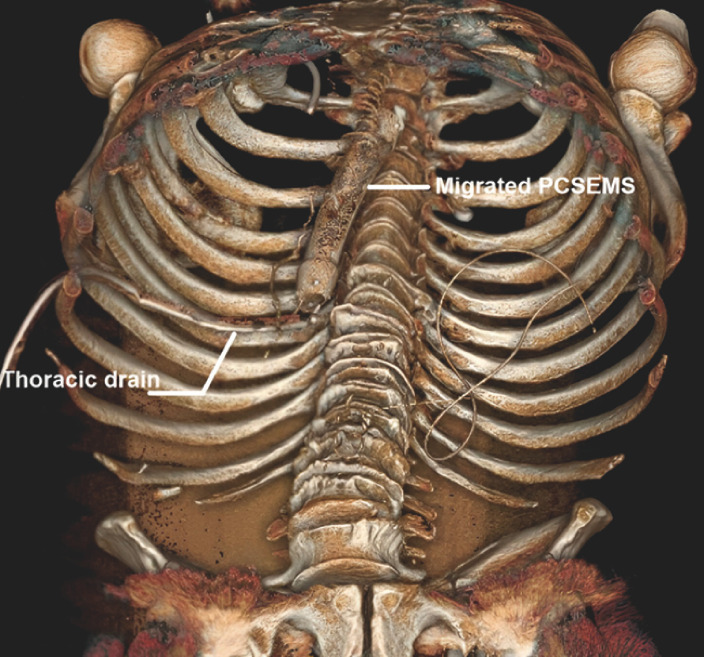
3D reconstruction from the initial computed tomography scan showing that the stent has migrated into the mediastinal cavity through the anastomotic fistula orifice.
